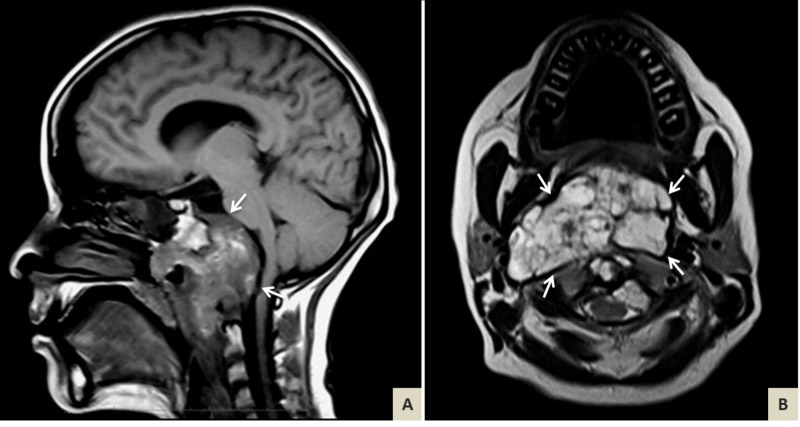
Brain magnetic resonance imaging (MRI) prior to surgery. (A) T1-weighted sagittal and (B) T2-weighted axial MRI showing heterogeneous enhancement with honeycomb appearance of a poorly defined spheno-occipital mass invading and eroding the clivus (white arrows). Features are consistent with areas of tumor hemorrhage and calcification. The pons and medulla are displaced.
